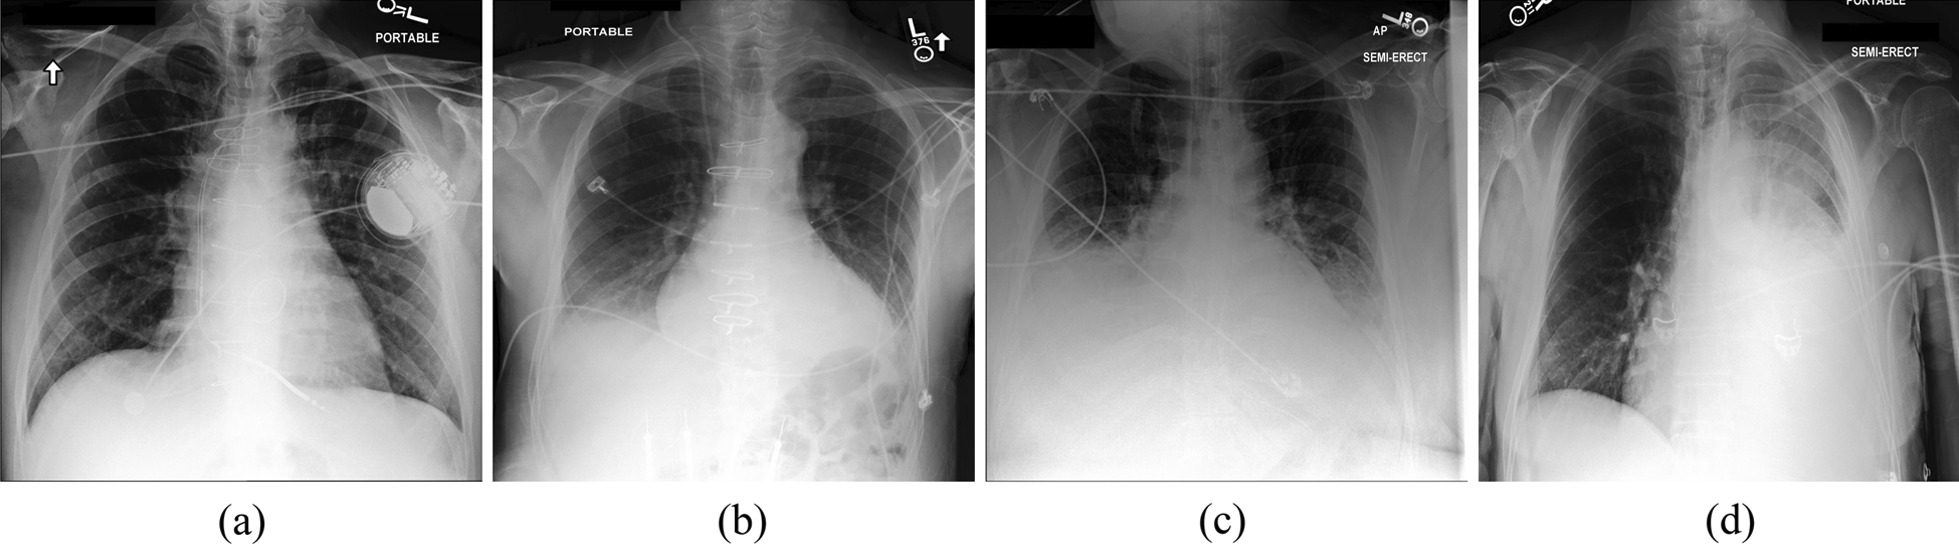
Examples of X-rays of pleural effusions of varying severity. a No effusion; b Small effusion; c Moderate effusion; d Large effusion
